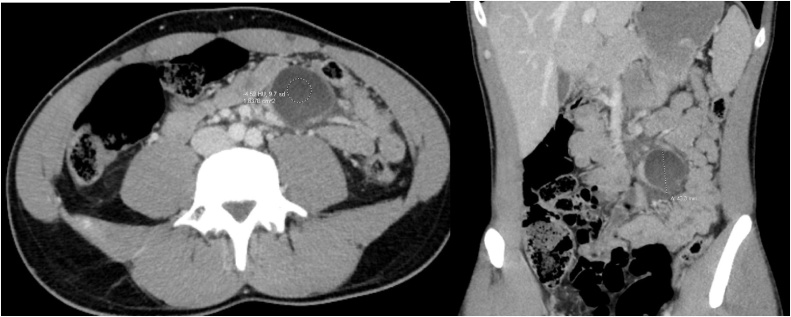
Computed Tomography of abdomen and Pelvic with IV contrast demonstrating left mid-abdominal mesenteric fluid collection near root of mesentery measuring up to 4.3 cm in largest dimension.
